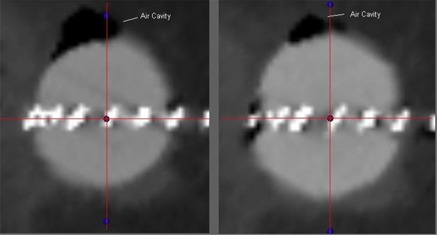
Pre‐ and post‐manipulation images of patient 2. The images are multi‐planar reconstructions of the computed tomography scan of the implant. The image on the left is the implant in its postoperative state. The image on the right is the implant after a net addition of 10 cm^3^ to the balloon volume. The size of the air pocket has been reduced, with an acceptable 0.25 mm increase in asymmetry.
